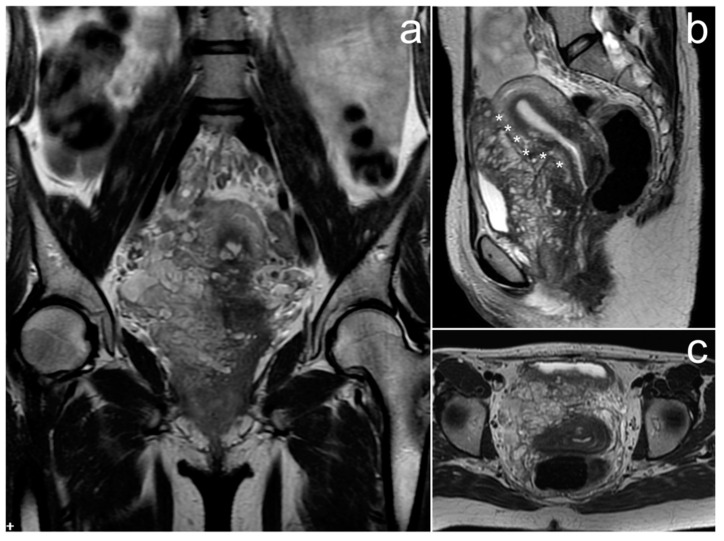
The pelvic MRI in Case 2 (a–c). The asterisks in (b) indicate infiltration of the anterior uterine wall and anterior cervical surface by plexiform neurofibroma. (a–c)—FSE/T2.
